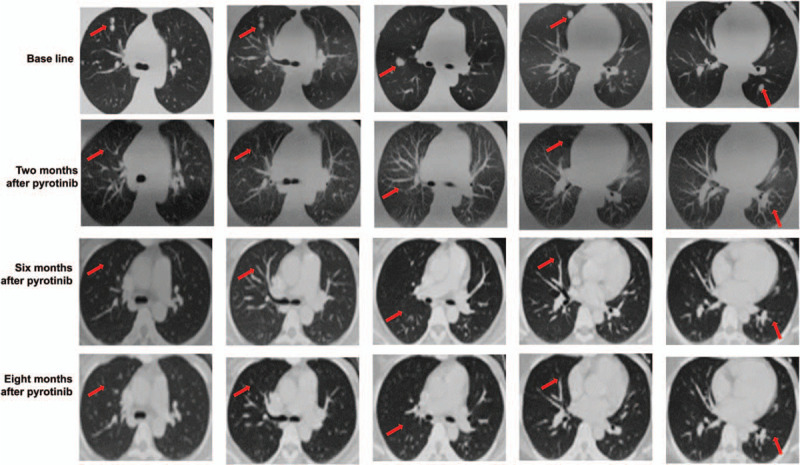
The computed tomography images (CT) of the lung before and after pyrotinib treatment. Red arrows represent the tumors.
